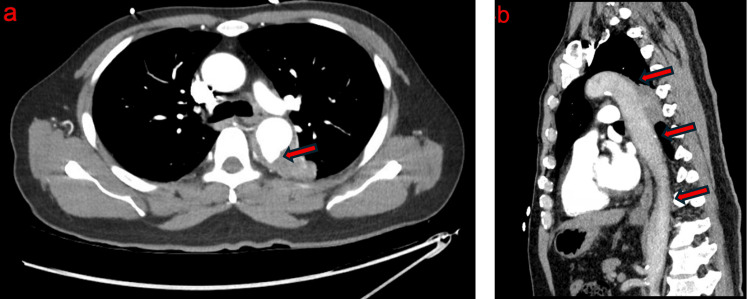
Penetrating aortic ulcer (PAU) of the descending thoracic aorta in the absence of significant atherosclerotic disease (a) Axial contrast-enhanced CT demonstrating a PAU localized to the dorsal surface of the proximal descending thoracic aorta, measuring 1.3×1.5×1.4 cm and a surrounding non-opacifying component measuring 4.2 cm (red arrow). (b) Sagittal non-contrast CT demonstrating the thoracic aorta with minimal atherosclerotic calcification and no significant plaque burden (red arrows).
